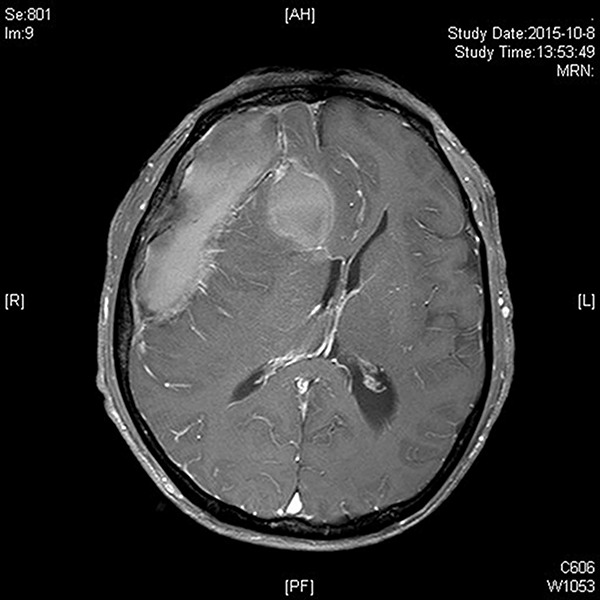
Admission magnetic resonance imaging of the patient.
